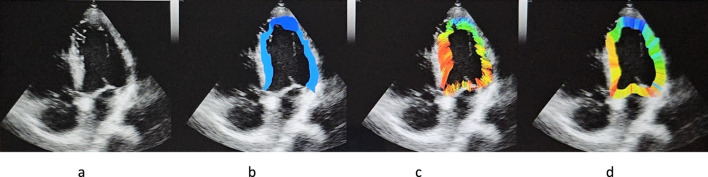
(a) Echocardiography of the left ventricle, (b) determination of the left ventricle contour, (c) displacement of the speckles, (d) projection of displacement along the radius launched from the left ventricle gravity center.
